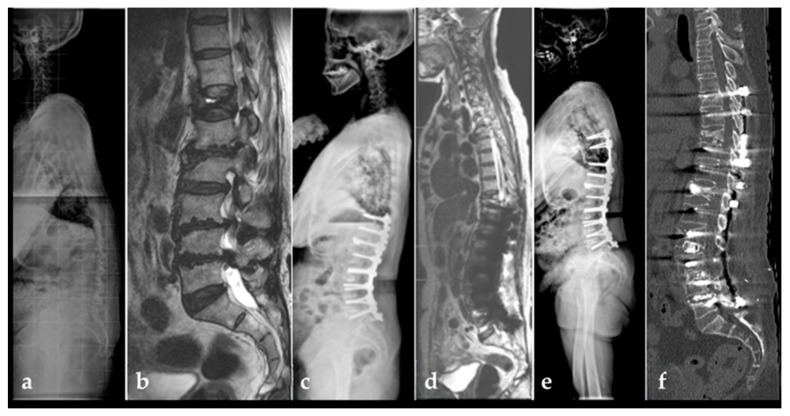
Representative case of proximal junctional failure. (a) Preoperative standing lateral radiograph of a 73-year-old female patient with degenerative lumbar scoliosis and sagittal imbalance, demonstrating an SVA = 105.2 mm; PI = 75.1°; TK = 13.1°; LL = −12.5°; PT = 47°; SS = 28.1°; PI-LL = 87.6°, DJA = 15°; and PJA = −4.0°. The modified Weiner grade for the L5–S1 disc was grade 0. (b) Preoperative magnetic resonance imaging demonstrating Pfirrmann grade C degeneration at L5–S1 with multilevel spinal stenosis. (c) Immediate postoperative standing lateral radiograph showing fusion from T10 to L5 with partial correction of sagittal alignment in SVA = 18.7 mm; PI = 75.6°; TK,14°; LL = −48.3°; PT, 41.2°; SS, 36.6°; PI-LL, 27.3°, DJA,15° and PJA −4.0°, indicating residual undercorrection. (d) Magnetic resonance imaging obtained four months postoperatively demonstrating a fracture at the upper instrumented vertebra plus one level (UIV+1), consistent with proximal junctional failure. (e) Follow-up standing lateral radiograph obtained two years after revision surgery demonstrating restoration of sagittal alignment without progression of L5–S1 disc degeneration. (f) Computed tomography obtained 28 months after the index surgery confirming the absence of advanced degeneration at the L5–S1 segment.
